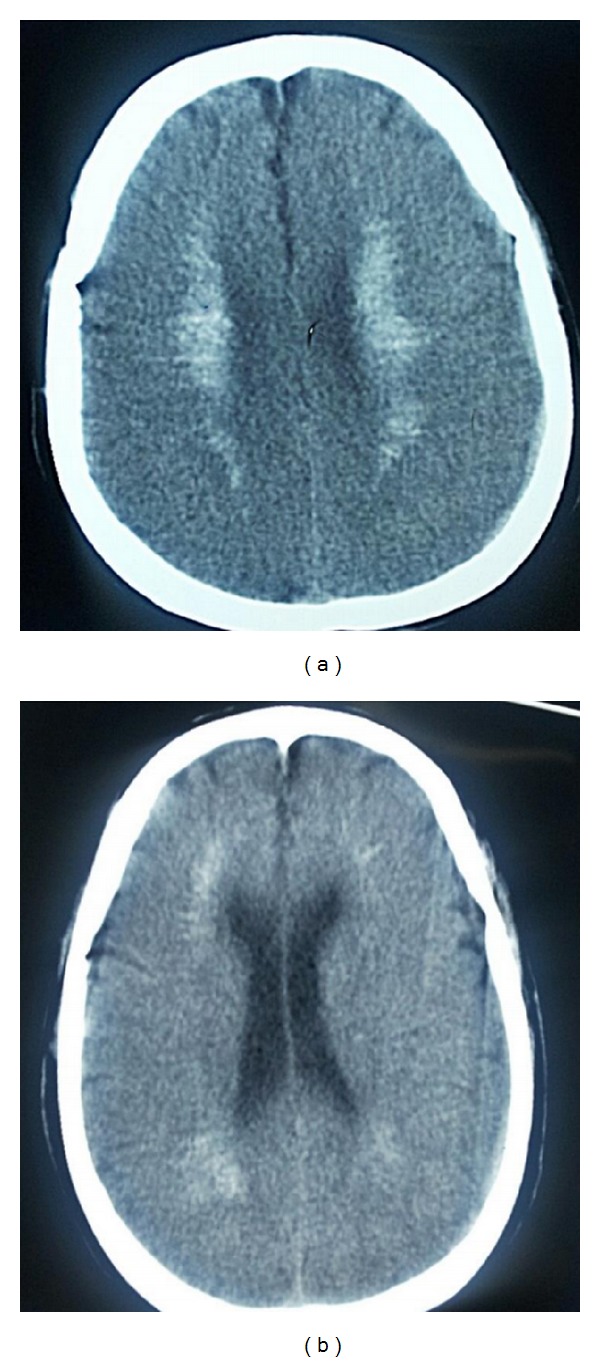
Noncontrast computed tomography of the brain showed intracranial calcification involving the basal ganglia, thalamus, and cerebral cortex on both the right (b) and left (a) sides.
